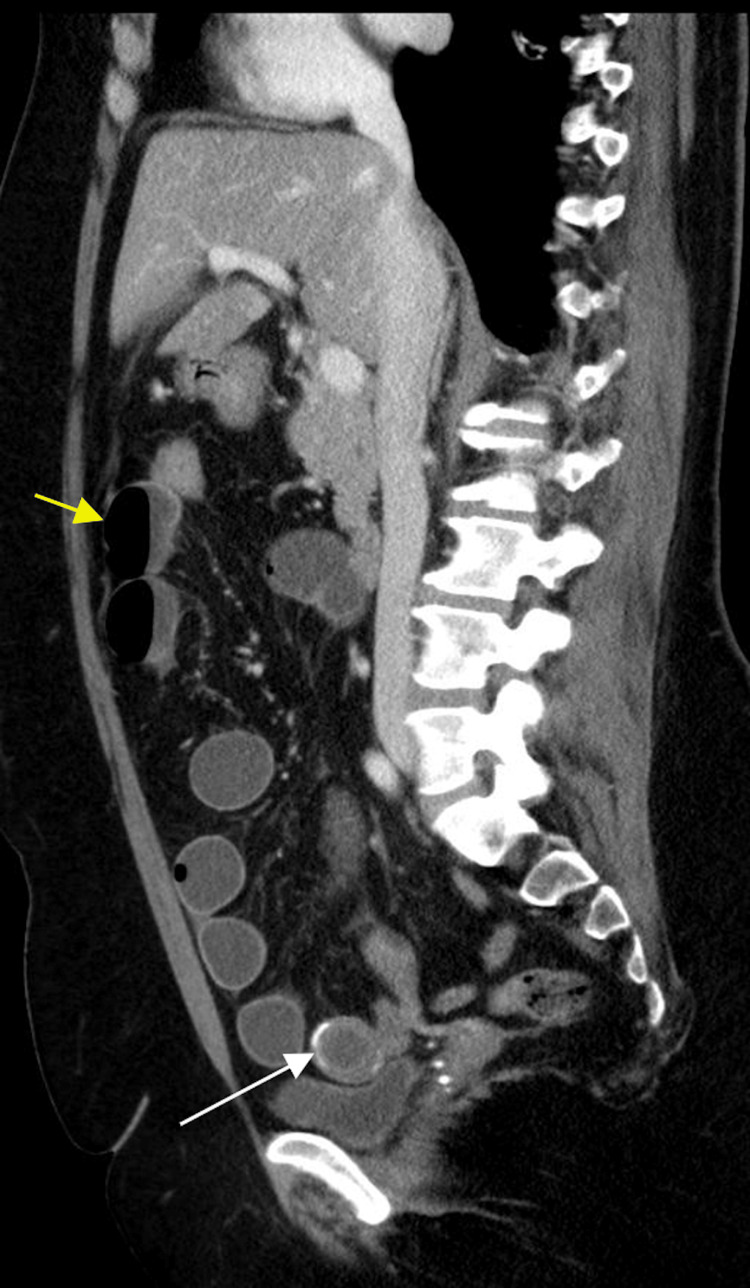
Contrast-enhanced CT of the sagittal view demonstrating a peripherally calcified gallstone (white arrow) within the terminal ileum and proximally dilated loops of the small bowel (yellow arrow) with air-fluid levels.
